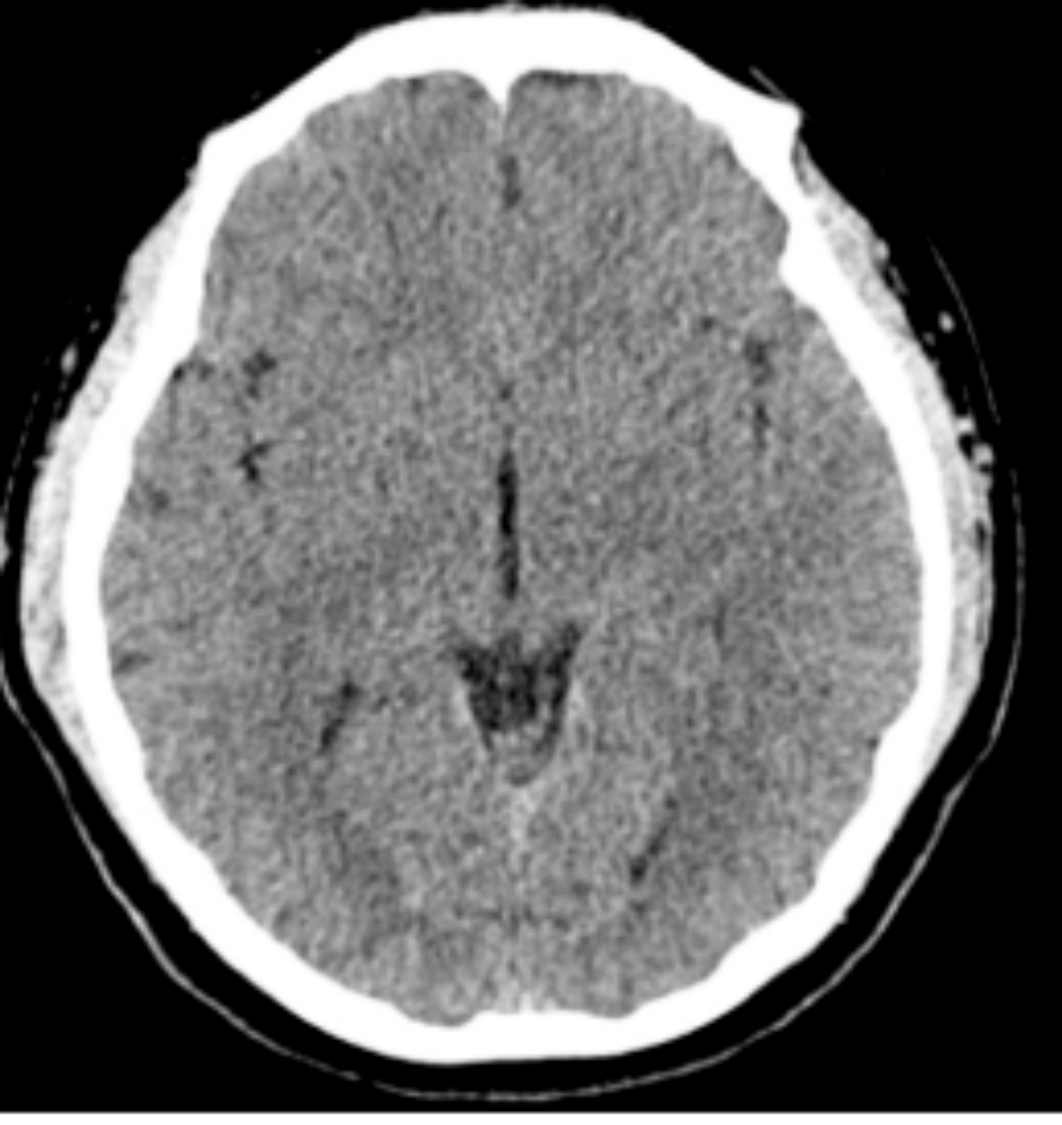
Computed tomography (CT) scan of the brain
